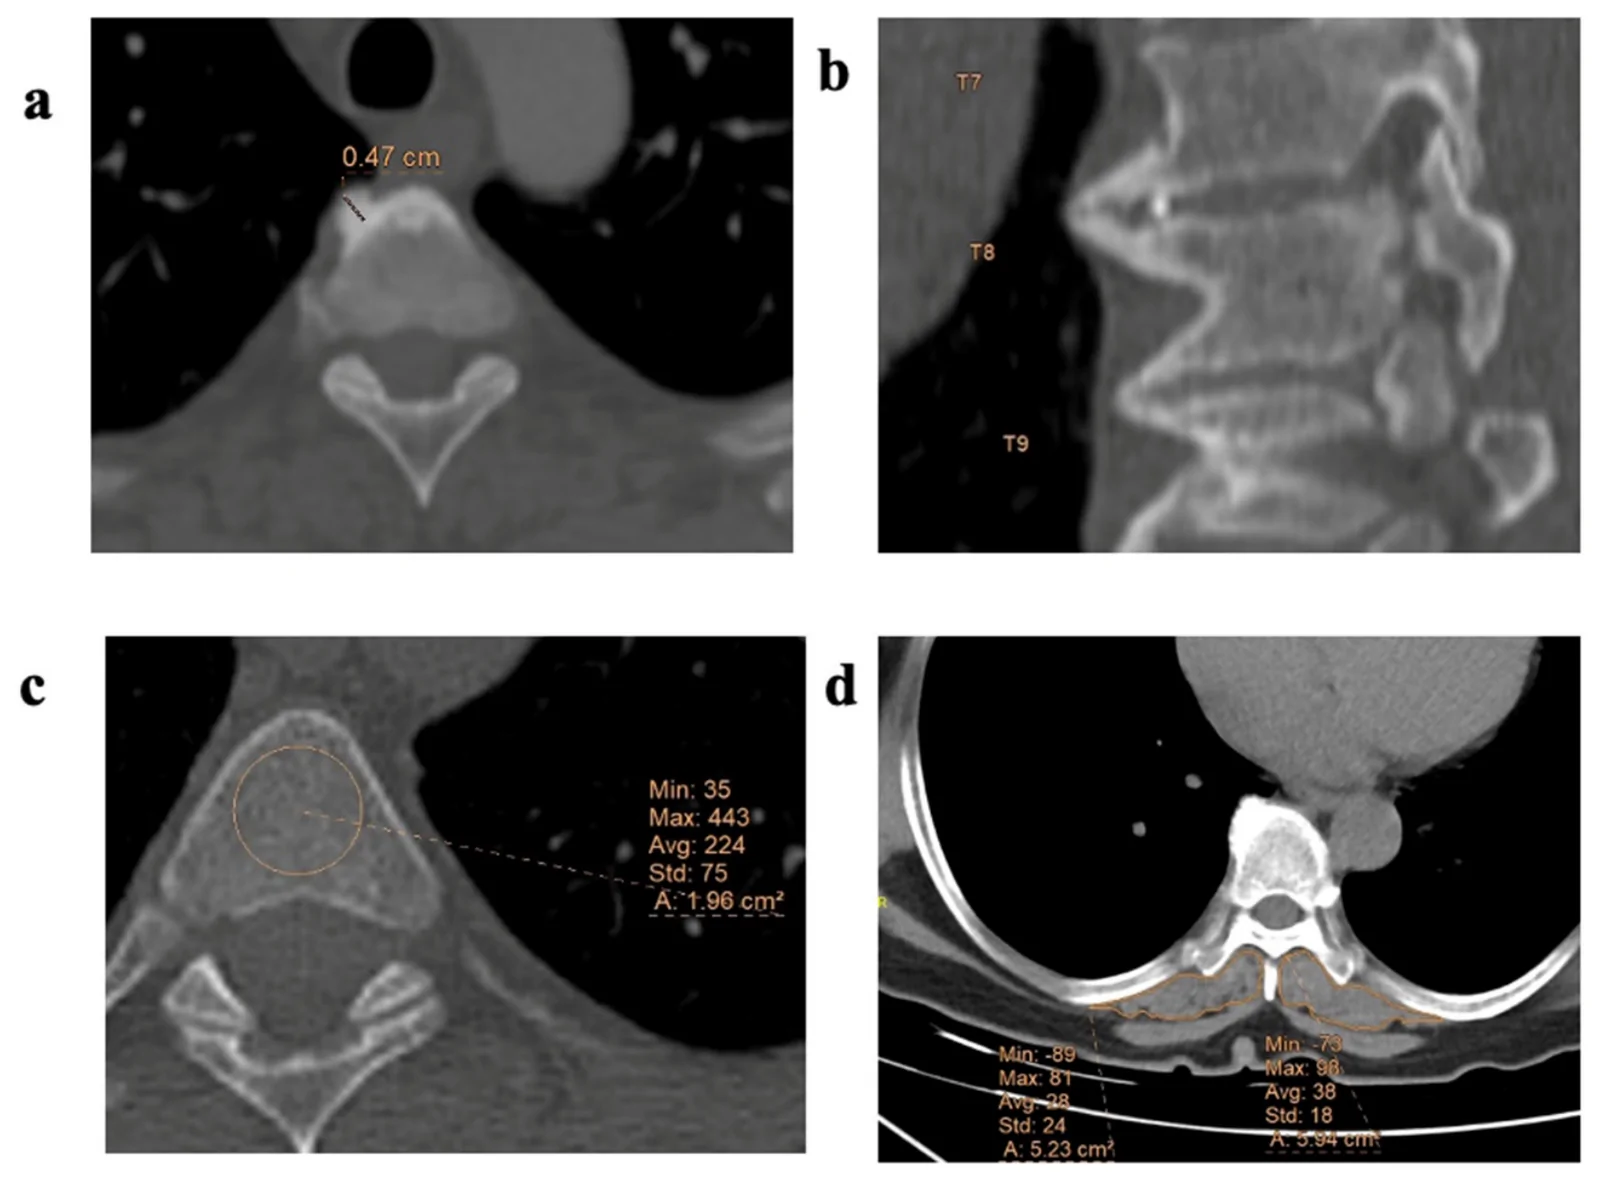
Representative CT images demonstrate the measurement parameters used in the study. (a) grade 3 according to the linear osteophyte classification; (b) grade 3 in the morphological osteophyte classification at superior and inferior end plate of T8 vertebra; (c) vertebral corpus bone attenuation measurement at the T8 level; (d) paraspinal muscle attenuation measurement at the T8 level.
